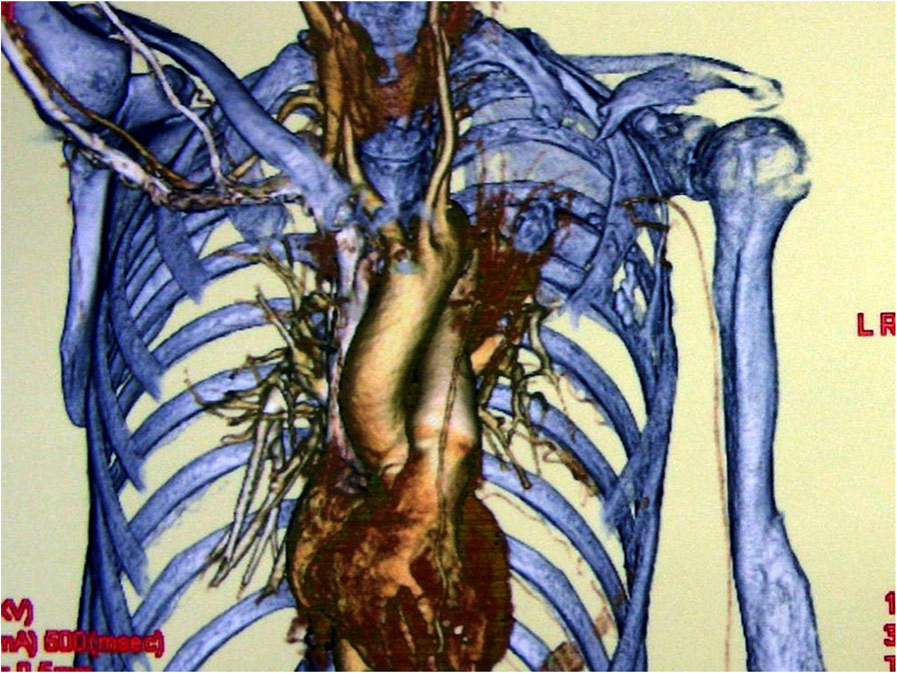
Preoperative computed tomography angiography. The subclavian artery was missing, and blood flow to the brachial artery was retrograde via the thoracodorsal artery to the subscapular artery
